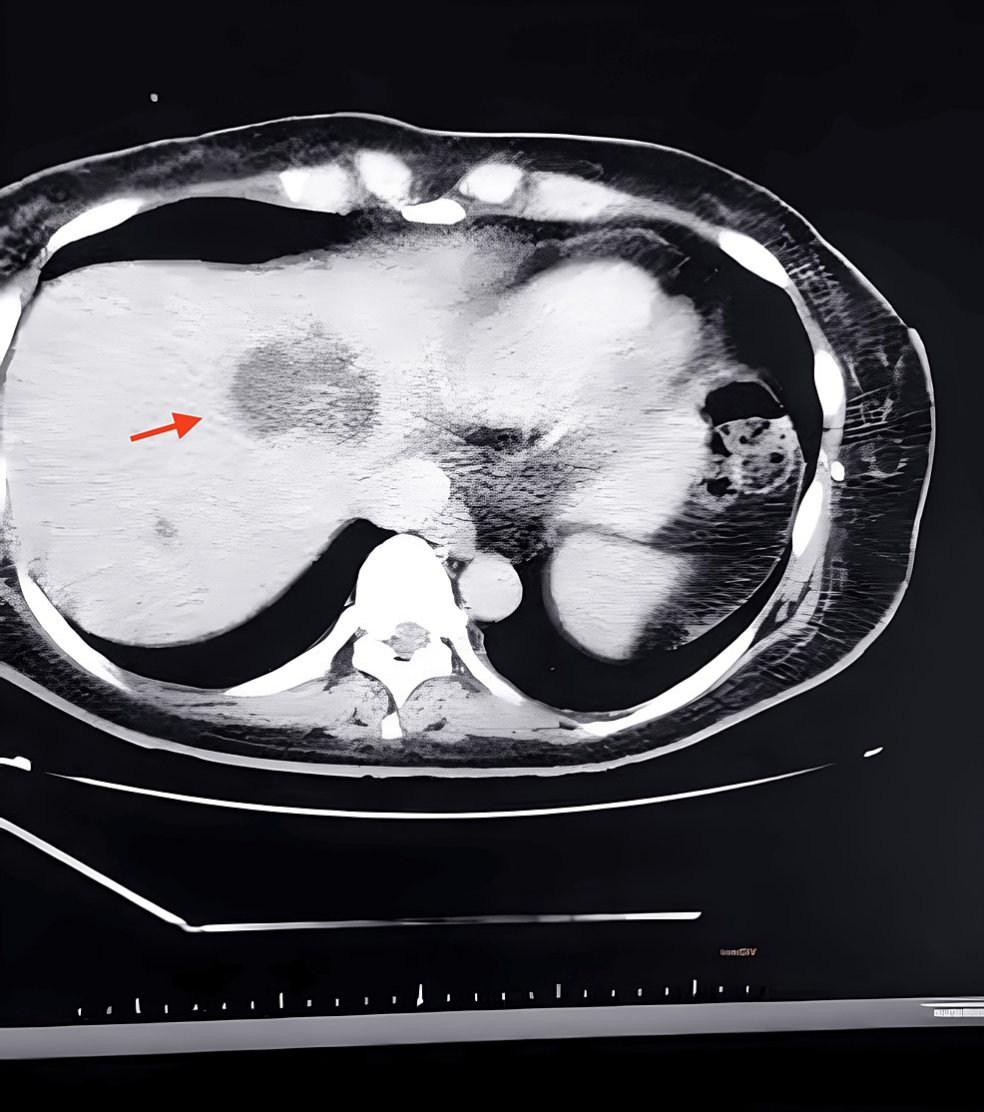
CT at Admission The red arrow shows the main hepatic lesion.
